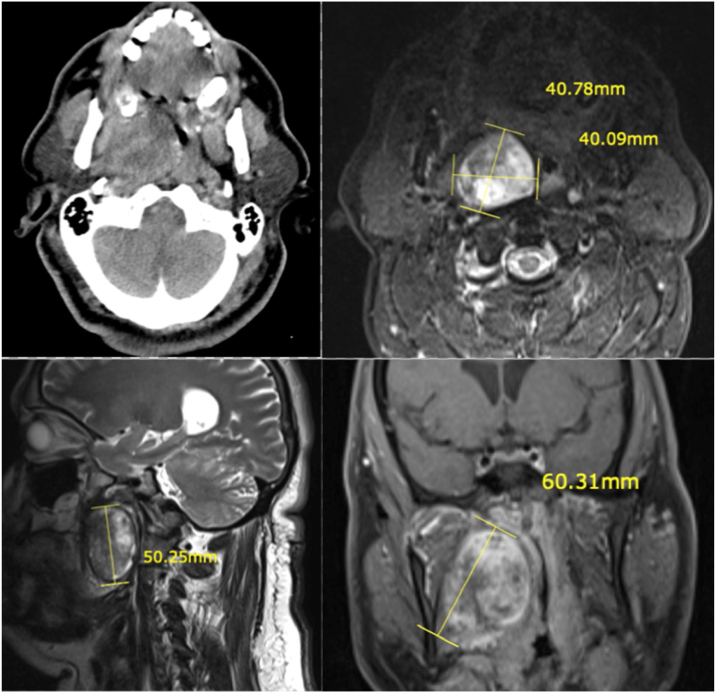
(a) Axial contrast-enhanced CT imaging. (b) Axial T2-weighted MRI. (c) Sagittal T2-weighted MRI. (d) Coronal plane T1-weighted fat suppressed and contrast-enhenced MRI.
